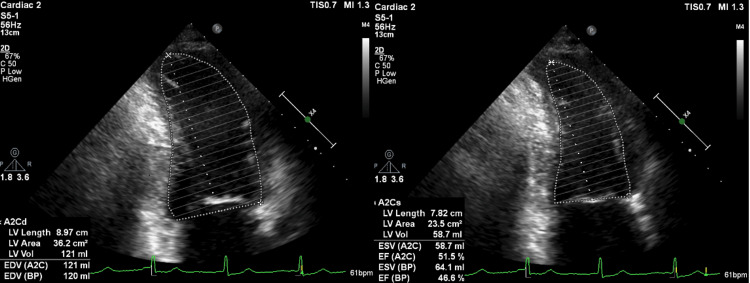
Follow-up transthoracic echocardiography on hospital day four. Transthoracic echocardiography in the apical two-chamber view was performed on hospital day four. The left image shows end-diastole and the right image shows end-systole. The calculated left ventricular ejection fraction (LVEF) was preserved at 46.6%, with no apparent valvular abnormalities or regional wall motion asynergy. Diffuse hypokinesis was noted, consistent with the clinical picture of transient cardiac dysfunction.
